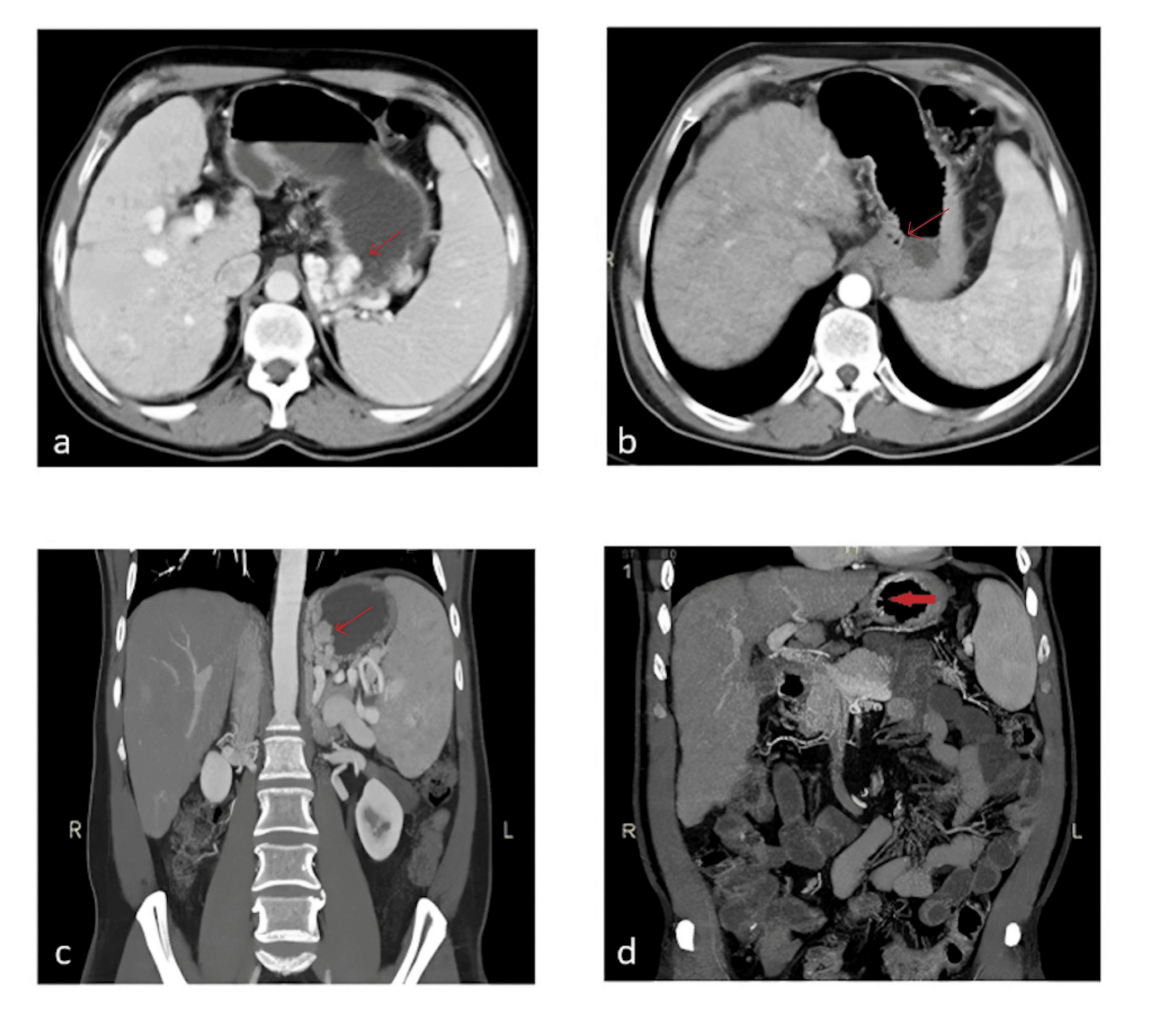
Contrast-enhanced computed tomography (CT) scan images of patients before and after balloon-occluded retrograde transvenous obliteration (BRTO) procedure (a) Contrast-enhanced CT (CECT) scan showing gastric fundal varices (arrow). (b) Post-BRTO CECT axial image showing total obliteration of the gastric fundal varices (arrow). (c) Coronal reconstruction of the CECT scan showing the gastric fundal varices (arrow). (d) Post-BRTO procedure CECT coronal image showing total obliteration of the gastric fundal varices.
